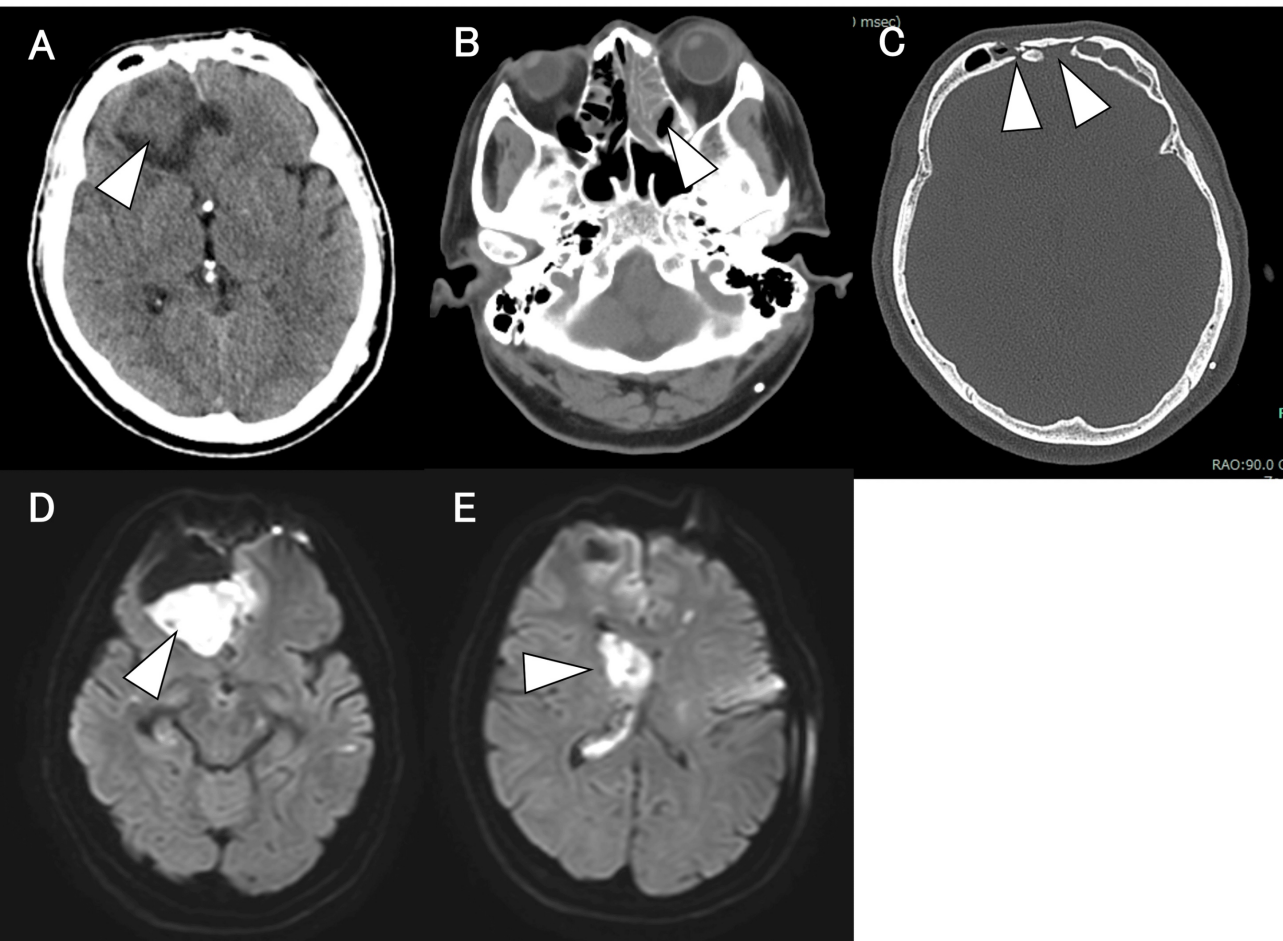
Imaging findings in Case 2 before transventricular endoscopic removal A: Head CT revealing a hypodense area with a partially hyperdense component in the right frontal lobe (arrowhead). B: Head CT showed sinusitis (arrowhead). C: Head CT confirming that the frontal sinus was patent (arrowhead). D and E: Brain MRI-DWI revealing high signal intensity areas in the right frontal lobe and right lateral ventricle, suggestive of ventriculitis and brain abscesses (arrowhead). CT, computed tomography; MRI, magnetic resonance imaging; DWI, diffusion-weighted imaging
